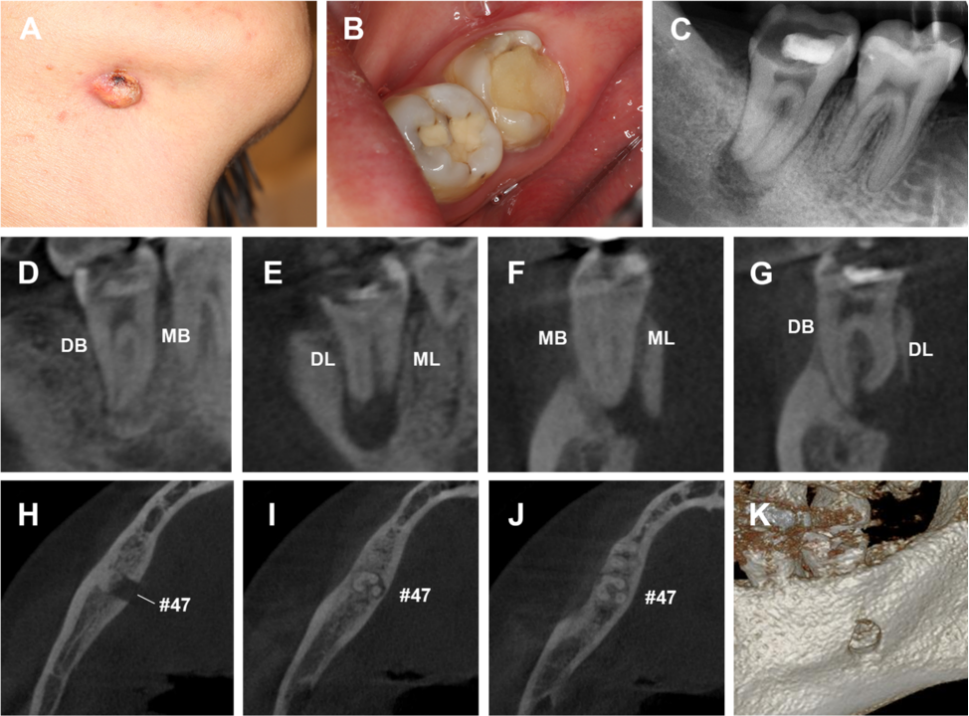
Preoperative clinical and radiographic examinations. (A) An extraoral cutaneous sinus tract presenting as an erythematous, smooth and non-tender nodule with crusting about 1 cm in diameter in the right submandibular region. (B) Preoperative clinical view of the right mandibular second molar with extensive glass-ionomer restoration. (C) A pretreatment radiograph of tooth #47 revealing obvious periapical radiolucency. CBCT imaging of the sagittal (D and E), coronal (F and G) and axial view (H-J) showing the extent of bone loss around the apexes and the distolingual (DL) root with curvature in the coronal third and additional buccal curvature from the middle third to the apical third. (K) The 3D - reconstruction of the CBCT showing the perforation of the lingual cortical plate. DB, distobuccal; MB, meisobuccal; DL, distolingual; ML, meisolingual.
